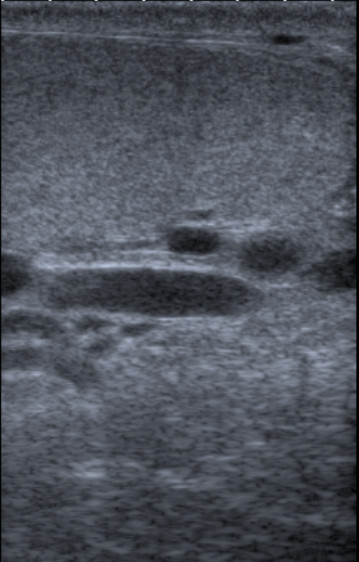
Ultrasound image – left testis focally normal.
